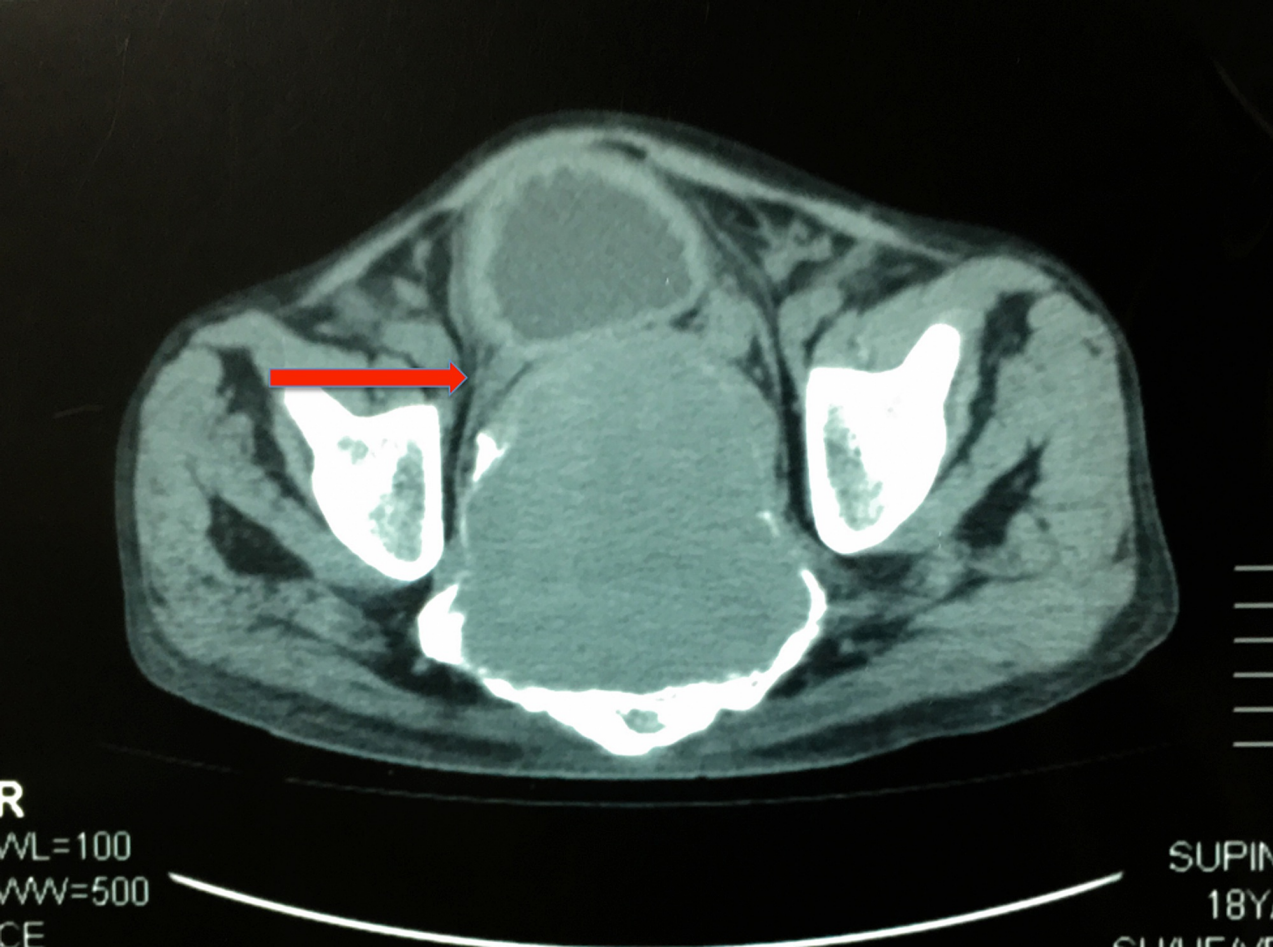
Axial CT image of a large expansile lytic lesion involving the midline sacrum compressing the bladder and ureters anteriorly CT: Computed Tomography
